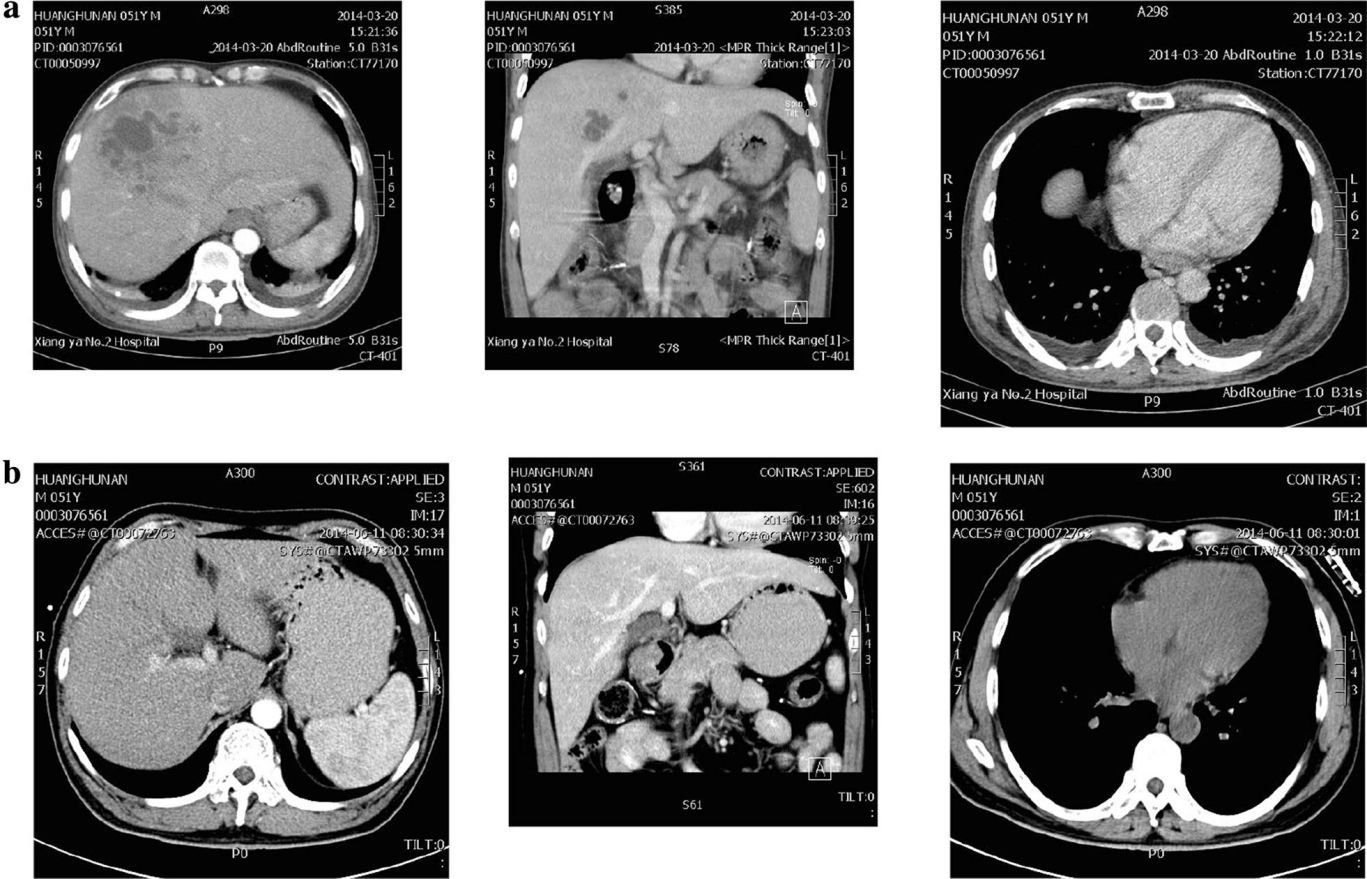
Computed tomography (CT) examination results of the teaching case. a Before CT-guided percutaneous aspiration and catheter drainage in the management of liver abscesses. b Three months after CT-guided percutaneous aspiration and catheter drainage in the management of liver abscesses
